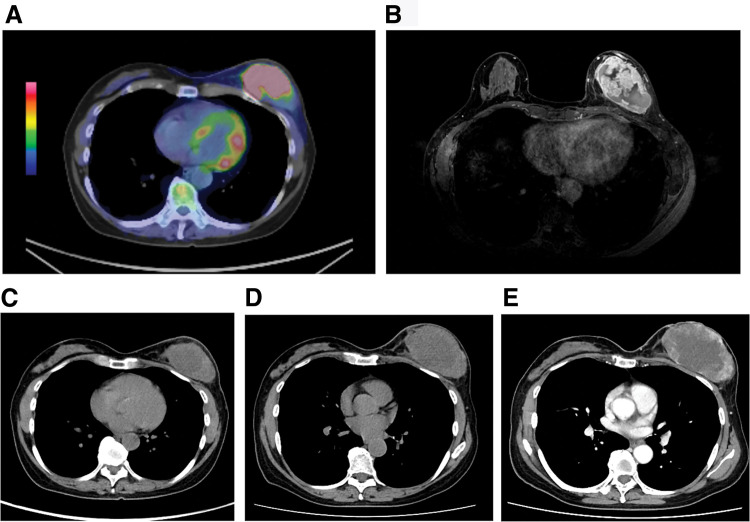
Serial radiological images before and during neoadjuvant chemo-immunotherapy. (A) PET/CT at presentation demonstrates a hypermetabolic left breast mass without evidence of distant metastasis. (B) Contrast-enhanced breast MRI at diagnosis reveals a well-defined enhancing lesion measuring approximately 7 cm, corresponding to the PET/CT findings. (C) CT at presentation demonstrates a left breast mass measuring approximately 7 cm. (D) CT prior to the initiation of chemo-immunotherapy shows interval enlargement to approximately 8.5 cm. (E) CT after 2 cycles of chemo-immunotherapy demonstrates further tumor enlargement to approximately 10 cm with ulceration, consistent with clinical progressive disease.
